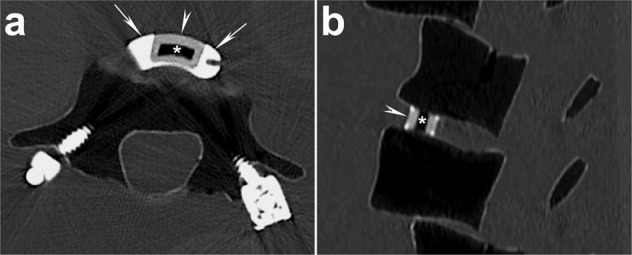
Appearance of titanium cage on dual-energy CT (DECT) imaged in a synthetic polyurethane foam/ hydroxyapatite lumbar spine model. a Axial and (b) sagittal reformatted 100 keV virtual monochromatic images (VMIs) reconstructed from a DECT scan of the spine show that the lattice portion has high density (arrowheads) but that is less than the solid titanium portions (marked by the arrows). The center is hollow (asterisks). Scan acquired using a rapid kV-switching CT scanner (Revolution CT, GE Healthcare)
